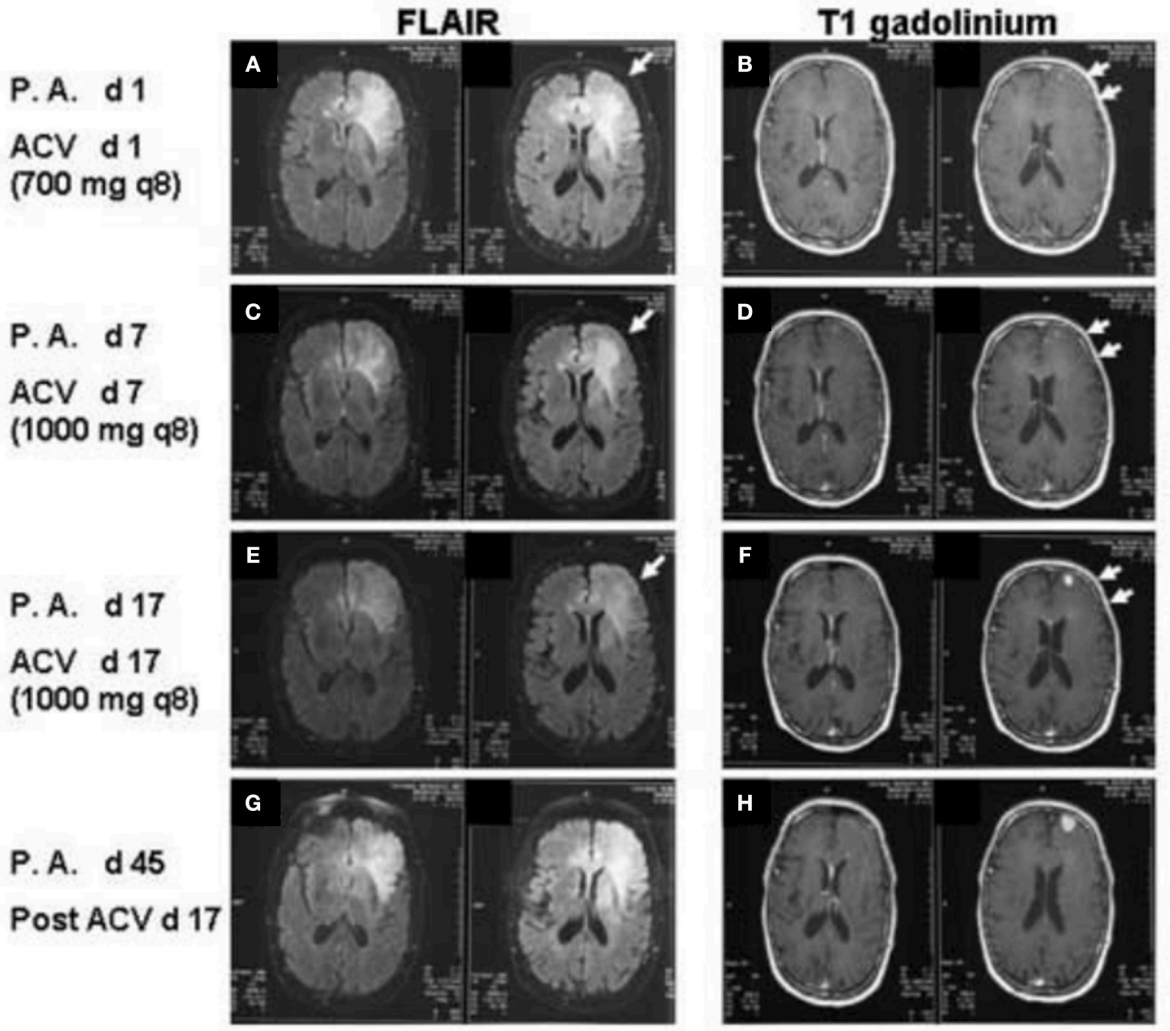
FLAIR images (A,C,E,F) T1 post-gadolinium images (B,D,F,G) of the patient at increasing days post-admission (P.A. d) and after administration of IV acyclovir (ACV d). Arrows in A, C, and E demonstrate decreasing FLAIR signal in the left frontal white matter from day 1 to day 17 of acyclovir administration. Double arrows in (B,D,F) demonstrate decreasing left frontal sulcal effacement and increasing size of the left frontal enhancing lesion. Increasing FLAIR signal and increasing size of the enhancing lesion on T1 post-gadolinium of the left frontal lobe are again noted on post-admission day 45 and post-acyclovir day 17 (G,H).
